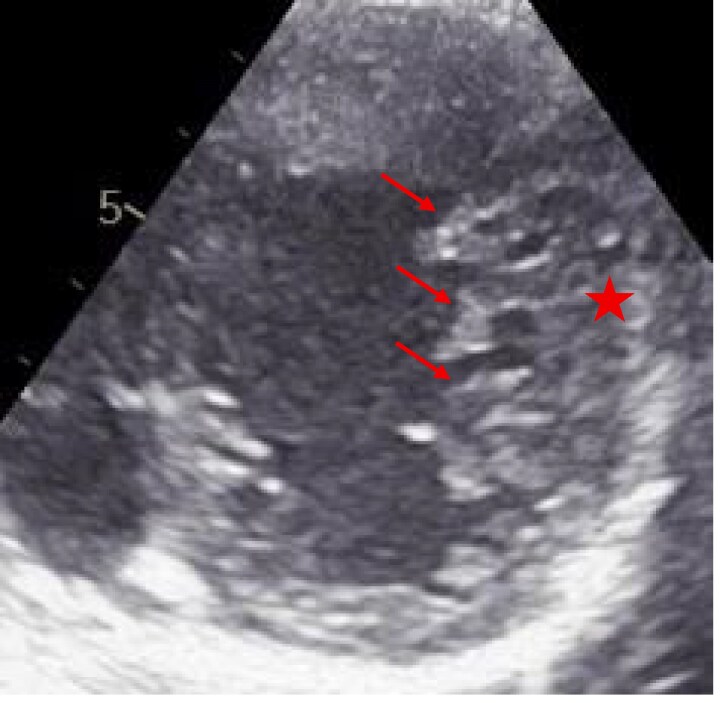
Short-axis echocardiographic view showing excessive trabeculations and deep intertrabecular recesses in the left ventricular myocardium, suggestive of left ventricular non-compaction. Red arrows highlight the regions of prominent trabeculation. The red asterisk indicates the compact myocardial layer.
